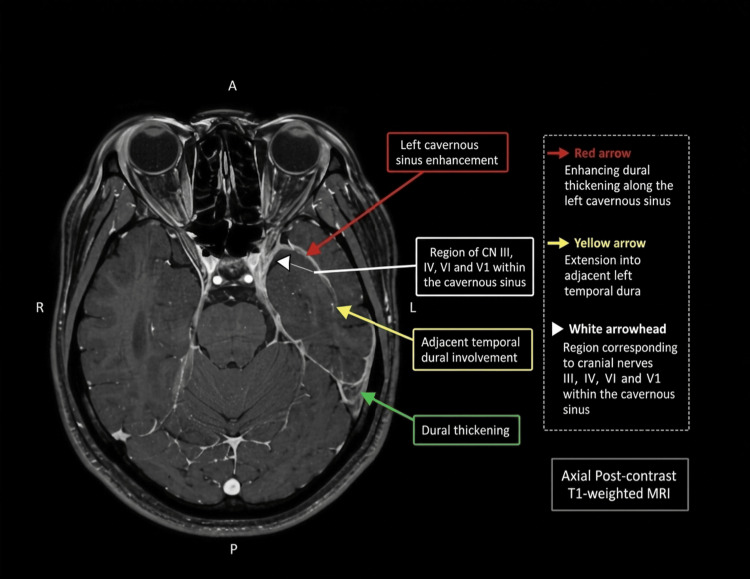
Contrast-enhanced MRI Brain, axial section, demonstrating hypertrophic pachymeningitis involving the left cavernous sinus. Axial post-contrast T1-weighted magnetic resonance imaging demonstrates abnormal enhancing dural thickening involving the left cavernous sinus (red arrow) with contiguous enhancement of the adjacent left temporal dura (yellow arrow). The lesion is located in the region traversed by cranial nerves (CN) III, IV, VI, and the ophthalmic division of cranial nerve V (white arrowhead), correlating with the patient’s ptosis, diplopia, ophthalmoplegia, and frontal sensory symptoms. The imaging findings are characteristic of hypertrophic pachymeningitis and, in conjunction with the exclusion of infectious, neoplastic, autoimmune, and vascular causes, supported the diagnosis of idiopathic hypertrophic pachymeningitis.
